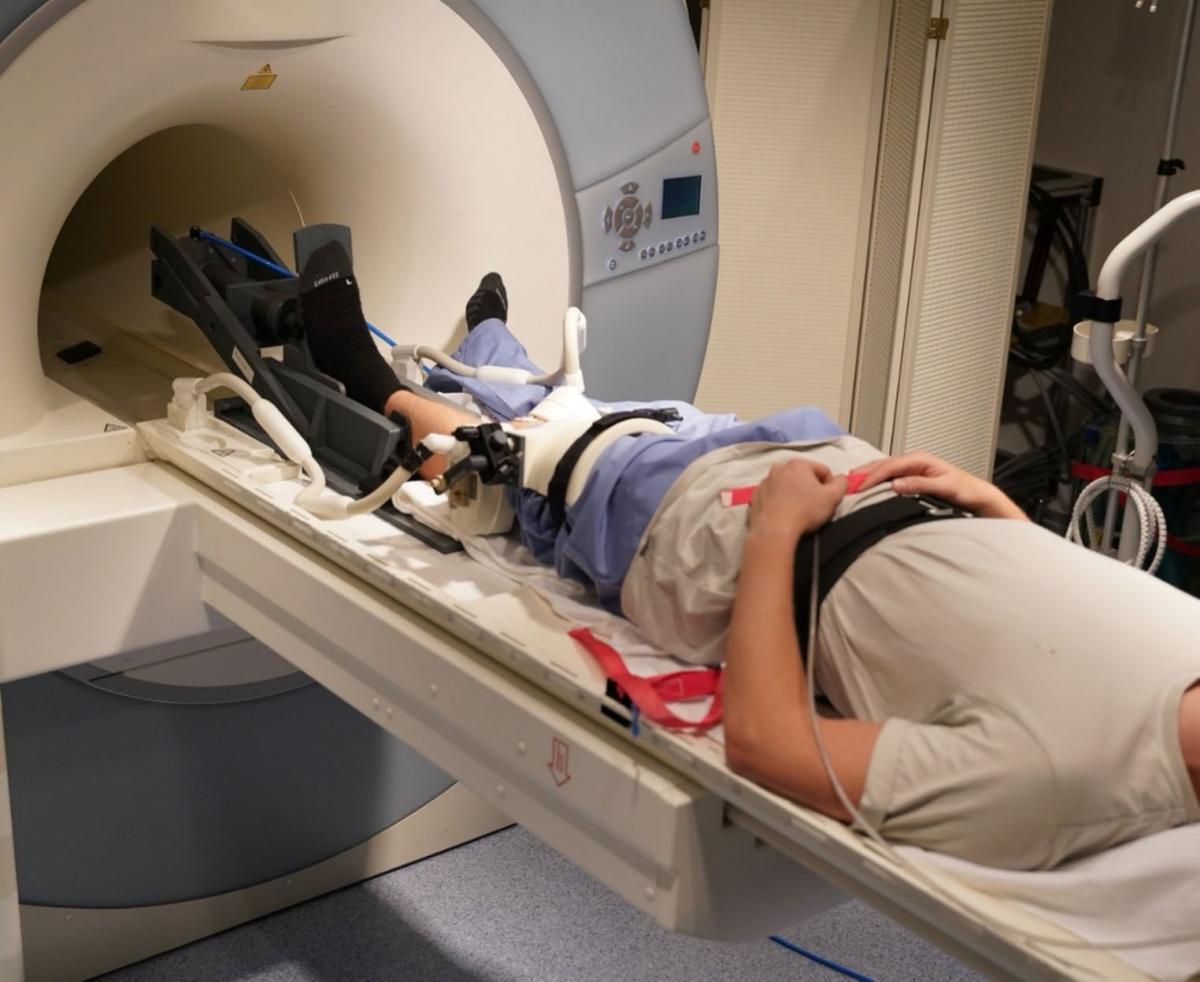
Setup of the MRI-compatible pneumatic loading device. The participant is positioned supine with the knee in full extension. To ensure isolated axial loading and prevent compensatory movements, the participant is firmly secured to the MRI table using fixation straps. The load is applied via a pneumatic footplate
